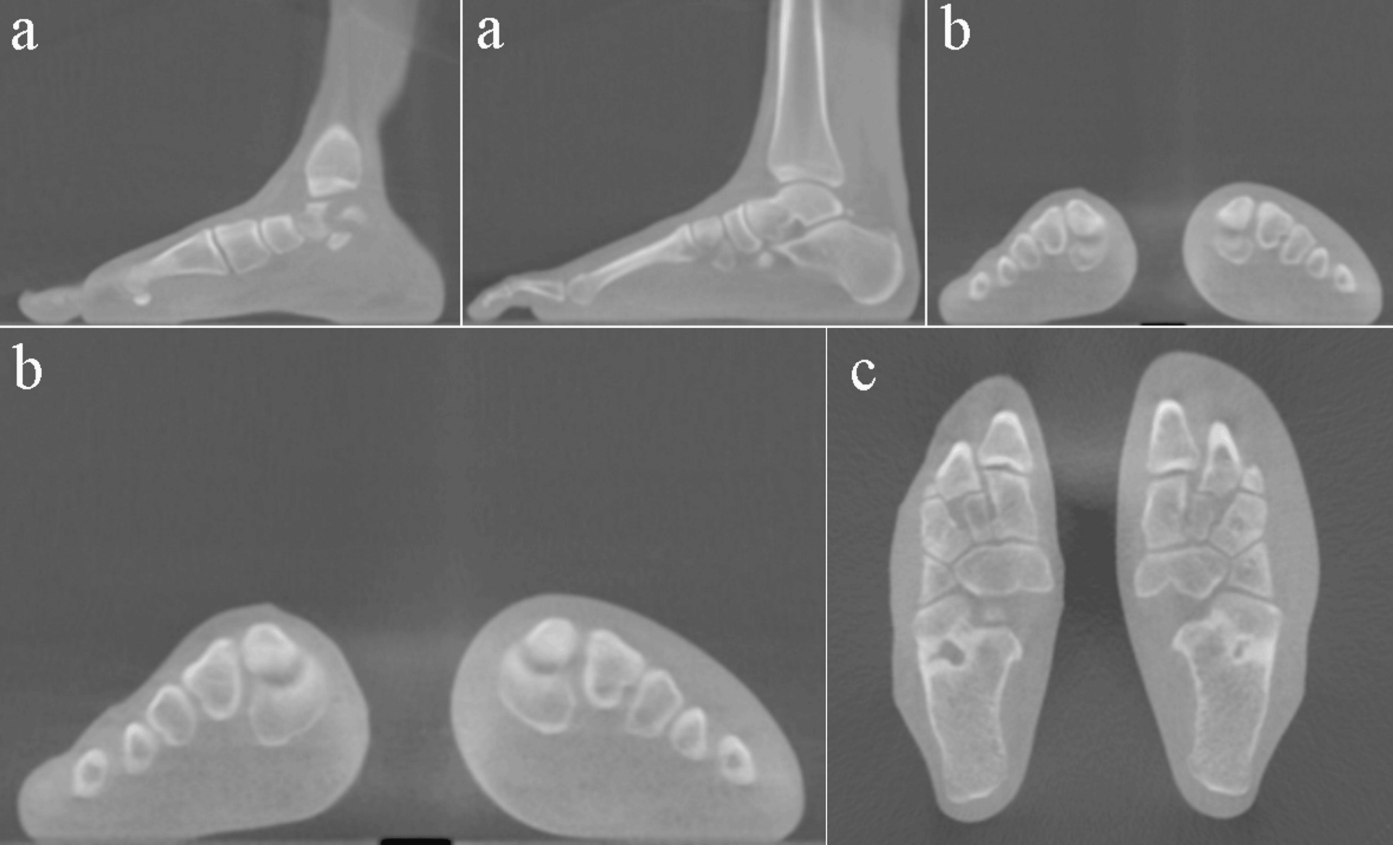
Bilateral WBCT images of the Lisfranc joint. Sagittal (a), coronal (b), and axial (c) views of the Lisfranc joint were included in the dataset to ensure capturing the joint space in a 3D manner. WBCT: weightbearing CT scan
